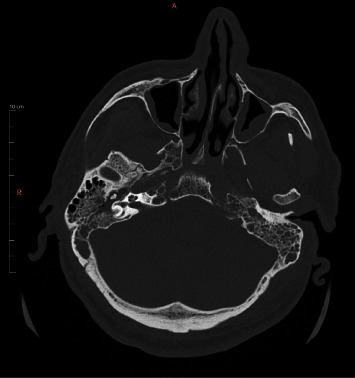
Brain CT showing fluid collection in both the middle ear and the mastoid cavities.
